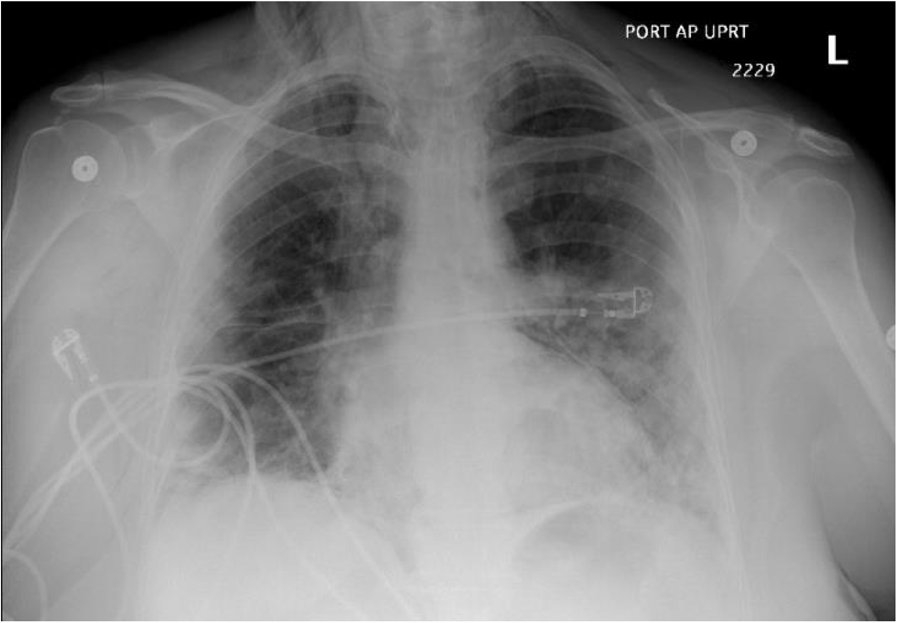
A frontal chest radiograph of Patient 1 showing interval development of pneumomediastinum, small pneumothoraces and subcutaneous emphysema
